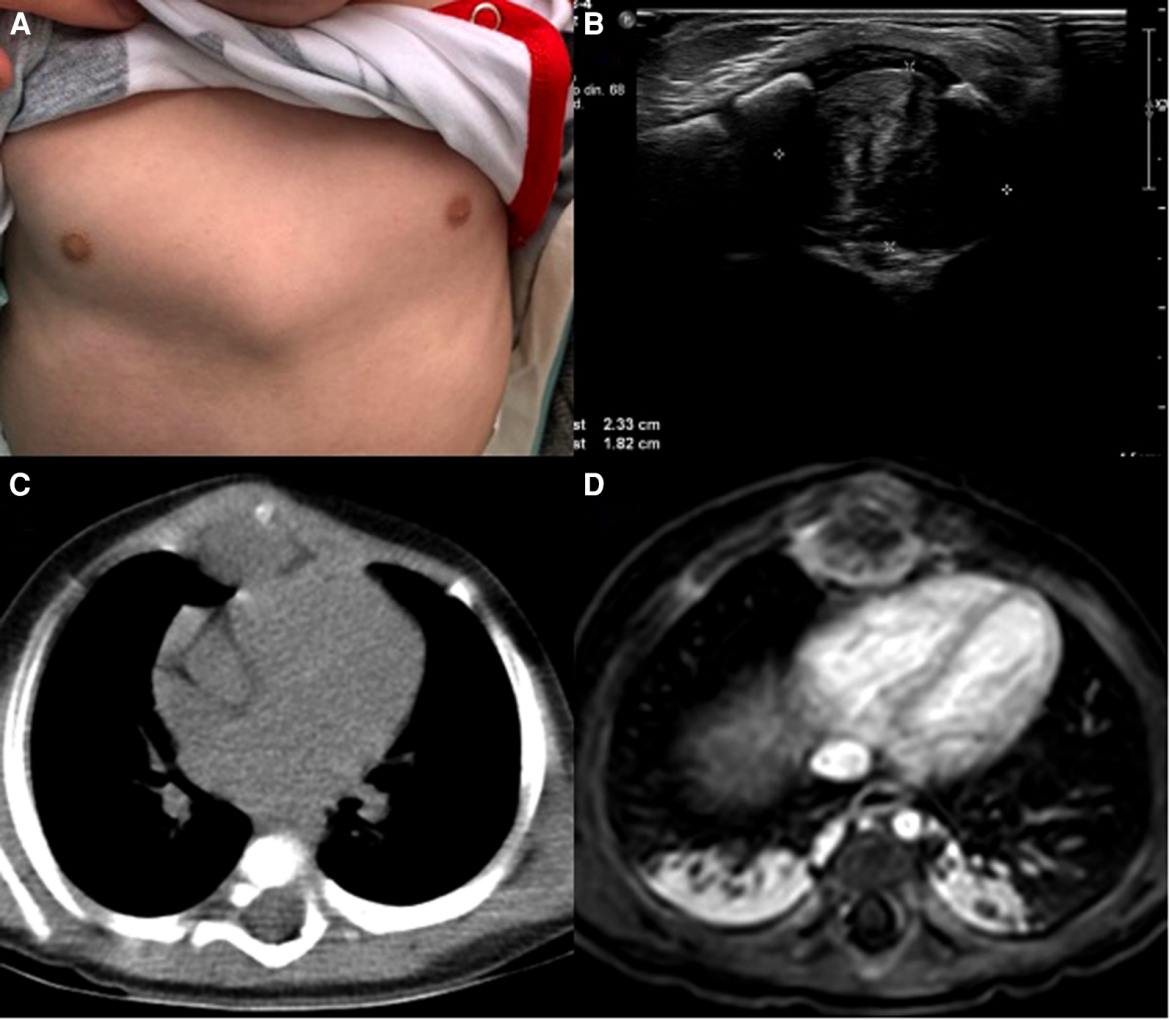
(A) chest mass, (B) ultrasound, echogenic mass in the anterior mediastinum. (C) CT, The mass is in close contact with the xiphoid process. (D) MRI, the mass is near the pericardium.
